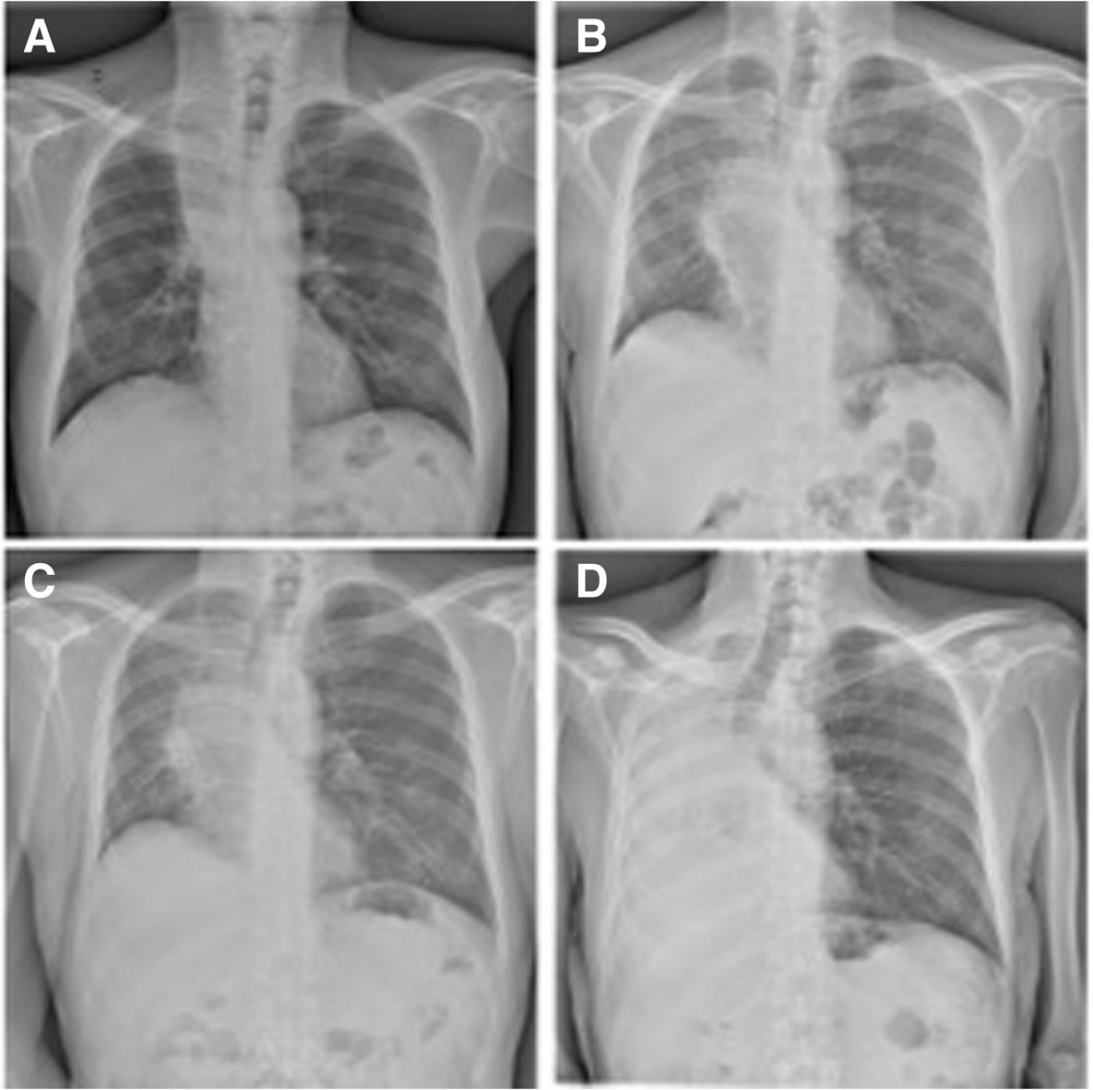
Chest x-rays showed pulmonary atelectasis involvement due to the cancer progression associated with four recurrent episodes of lung infection
